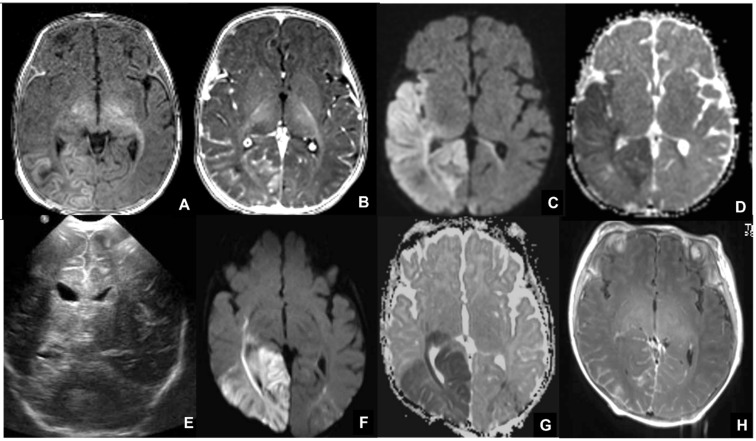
MRI of neonate affected by herpes virus type 1 (A–D) presenting with seizures and right hemiparesis, showing cortical-subcortical signal alteration on pre-contrast T1WI (A), contrast enhancement on post-contrast T1WI and diffusion restriction on DWI/ADC (C,D) in the right parietal-insular region. Neonate affected by herpes virus type 2 undergoing US, showing cortical-subcortical hyperechogenicity in the right posterior temporal and left parietal regions (E), and undergoing MRI, showing diffusion restriction on DWI/ADC (F,G) and contrast-enhancement in the abovementioned areas (H).
